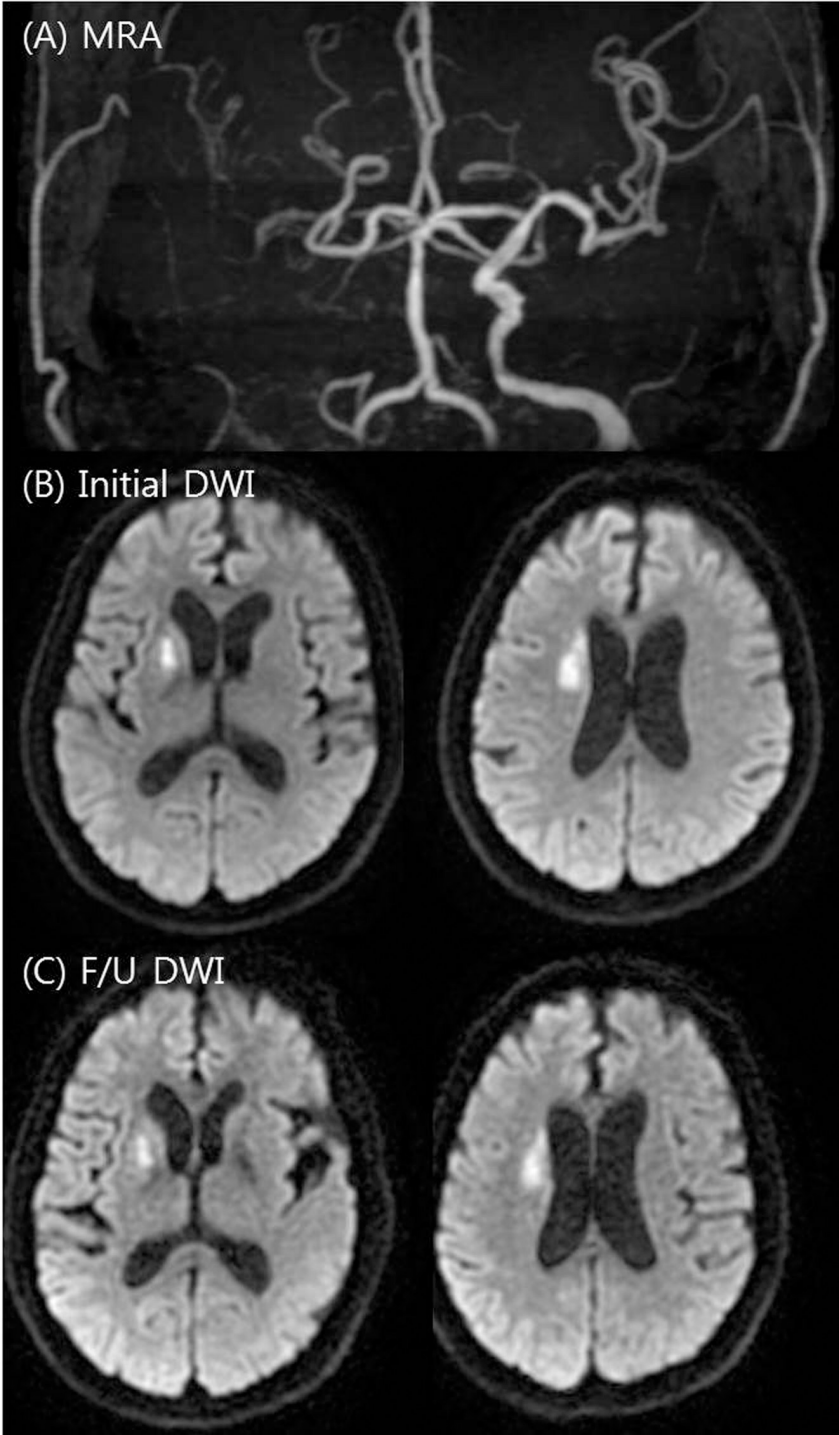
Example of a low percent insular ribbon infarction (PIRI) score. A 75-year-old man presenting 350 minutes after stroke onset with an NIHSS score of 1. MRI showed right internal carotid artery occlusion on time-of-flight intracranial MRA (A), with an ischemic lesion in the right putamen but a normal insula on initial DWI (B; PIRI score 0). On the follow-up DWI, no significant lesion changes were observed (C).
